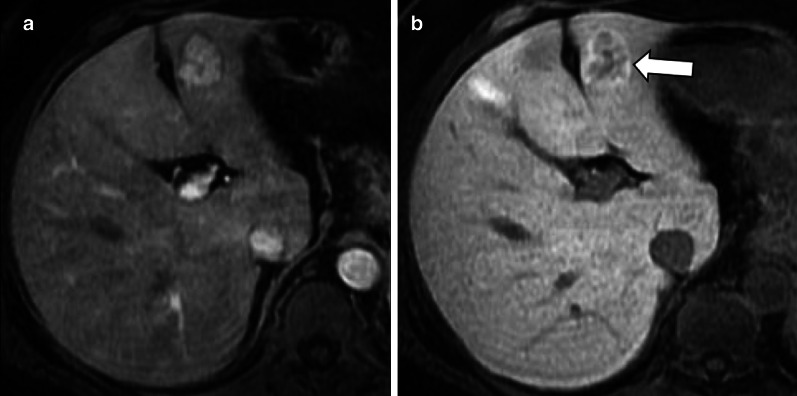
A 44-year-old woman with focal nodular hyperplasia. Gadobenate dimeglumine-enhanced MRI shows a focal nodular hyperplasia that is (a) hypervascular in the arterial phase and (b) demonstrates a hyperintense rim (arrow) in the hepatobiliary phase
